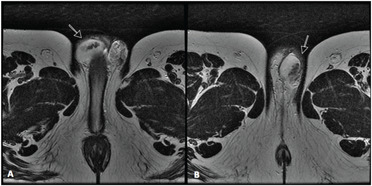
Magnetic resonance imaging (MRI) – Sagittal T2 images revealing low-sign right (A) and left (B) solid elongated serpiginous lesions in the testicular mediastinum (arrows).
